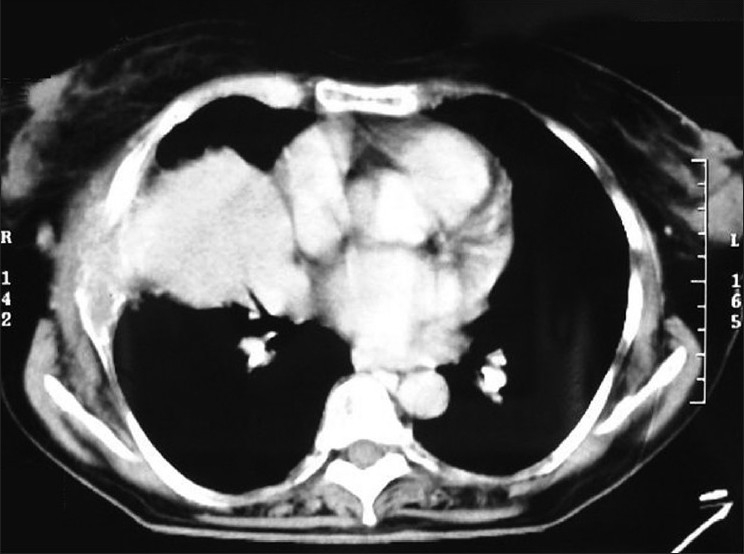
Computed tomographic scan of the thorax (CT thorax) revealing soft tissue mass in the right anterior chest wall with rib destruction and lytic lesions in lateral end of right clavicle and posterior end of fifth rib suggestive of metastaic deposits
